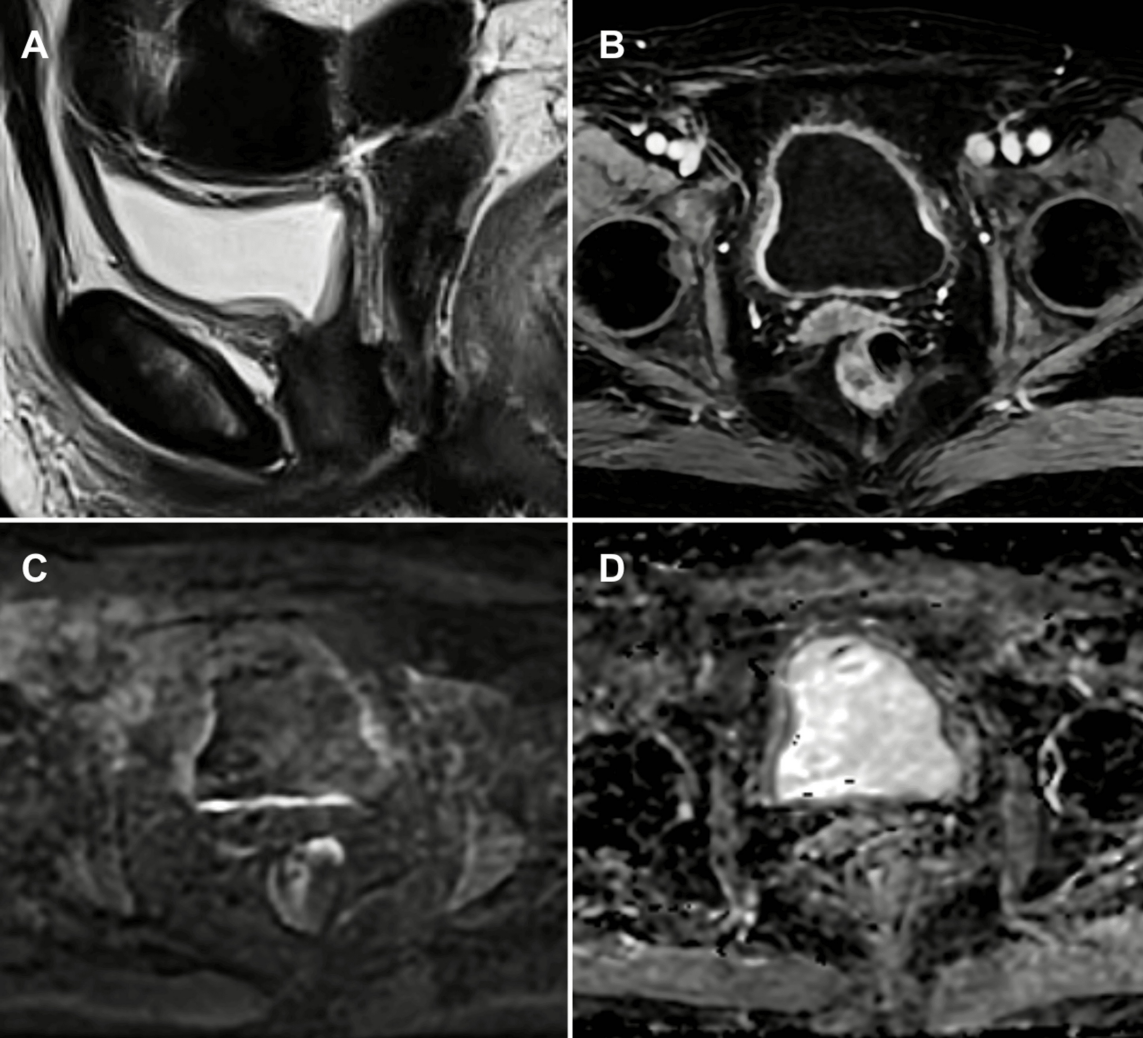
mpMRI of post-BCG bladder wall changes: distinguishing reactive inflammation from residual tumour (A) Sagittal T2-WI, (B) axial DCE imaging, (C) axial DWI, and (D) axial ADC map. mpMRI of a bladder tumour patient treated with TURBT followed by intravesical BCG instillation. The T2-weighted image demonstrates mucosal thickening resembling bullous oedema of the bladder wall. The DCE image shows diffuse enhancement of the bladder wall. Despite diffuse hyperintensity within the bladder wall on DWI and corresponding hypointensity on the ADC map, these features are interpreted as reactive changes related to recent treatment rather than tumour recurrence or residual disease. This case exemplifies the challenge of differentiating post-treatment inflammatory changes from viable tumour using mpMRI. Patient consent for publication was obtained. mpMRI: multiparametric magnetic resonance imaging; T2-WI: T2-weighted imaging; DCE: dynamic contrast-enhanced; DWI: diffusion-weighted imaging; ADC: apparent diffusion coefficient; TURBT: transurethral resection of bladder tumour; BCG: Bacillus Calmette-Guérin
